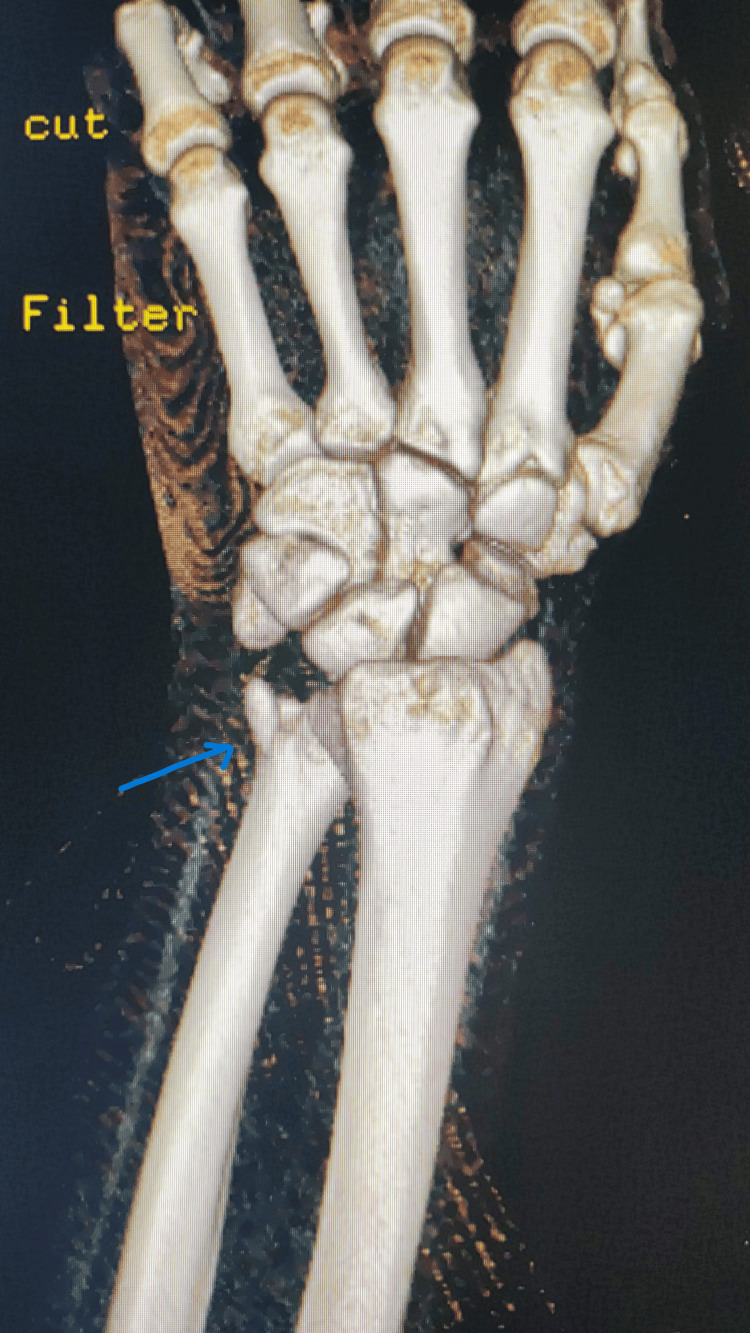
3D computed tomography Computed tomography 3D reconstruction demonstrating volar dislocation of the distal radioulnar joint. Blue arrow pointing to the ulnar styloid process. Figure created using Philips IntelliSpace PACS (formerly known as iSite) (Amsterdam, Netherlands).
